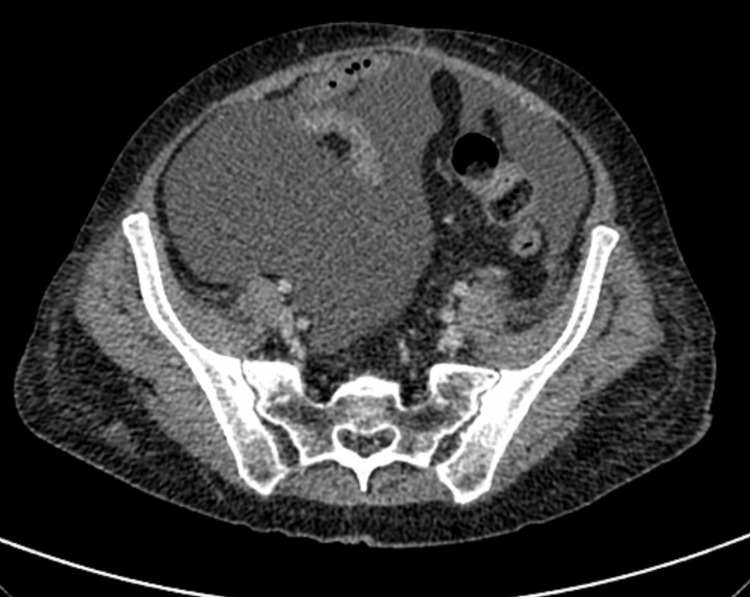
CT scan of abdomen, axial plane: free peritoneal fluid.
